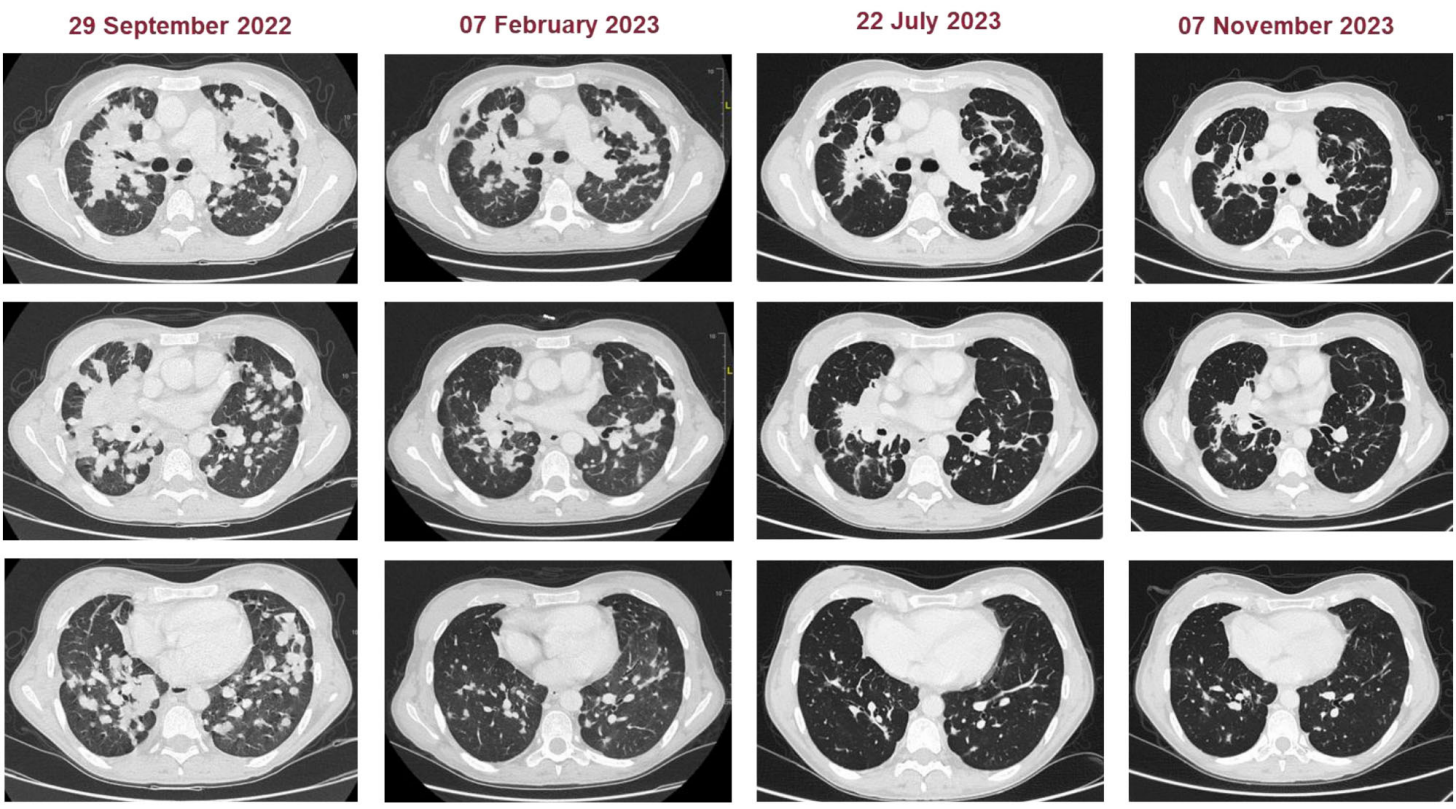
Representative CT images of patients at baseline and during the treatment (subsequently to chemoimmunotherapy, double chemotherapy, and maintenance chemotherapy).
